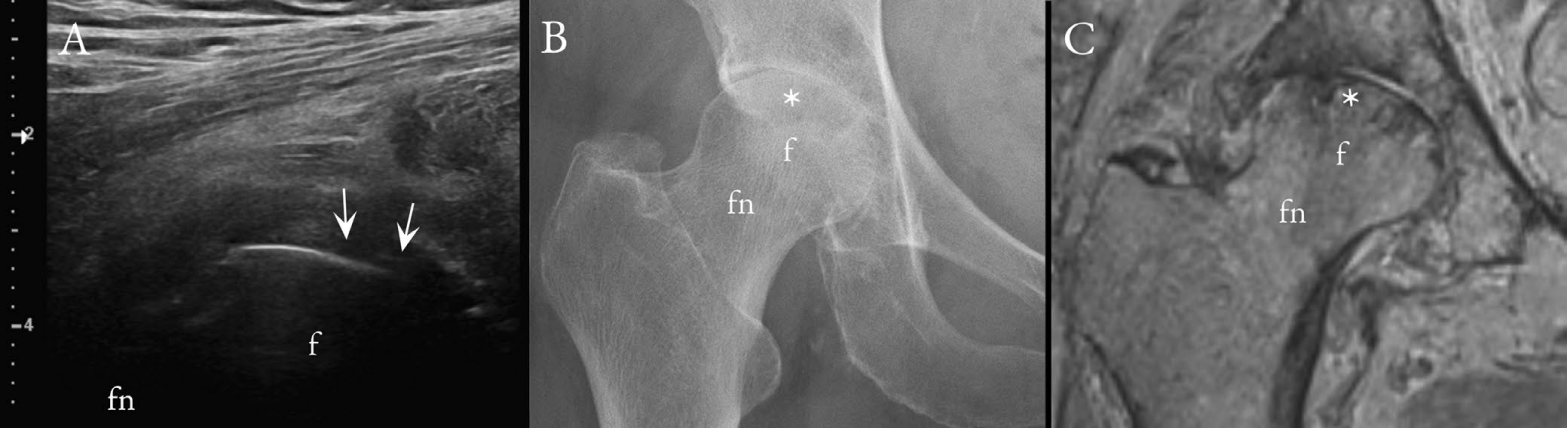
A 75‐year‐old woman with loss of normal femoral head sphericity and visibly flattened subchondral bone surface in longitudinal US (A). Anteroposterior CR (B) shows local subchondral lucency in the superior joint space (asterisk) and slight femoral head shape abnormality. Coronal PD‐weighted MRI (C) shows attrition‐associated subchondral cysts, sclerosis, and flattening of the femoral head. f, femoral head; fn, femoral neck.
